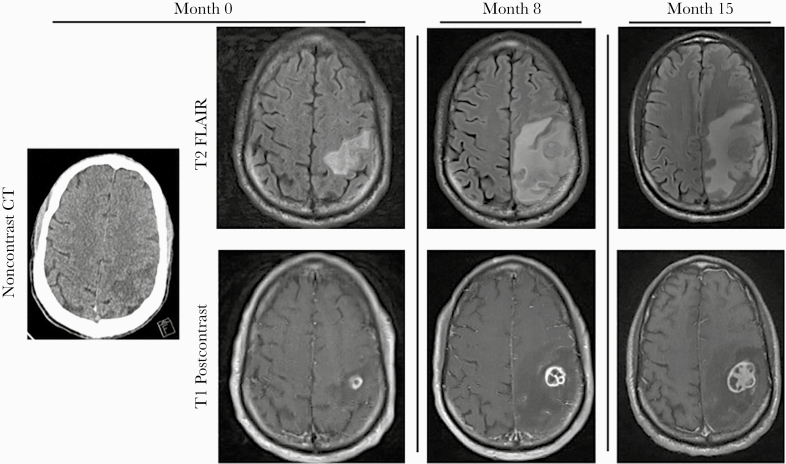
A, Noncontrast head CT, T2 FLAIR precontrast MRI, and T1-weighted postcontrast MRI from initial presentation. B, T2 FLAIR precontrast MRI and T1-weighted postcontrast MRI after discontinuing ART and MAC therapy. C, T2 FLAIR precontrast MRI and T1-weighted postcontrast MRI following completion of prednisone taper. Abbreviations: ART, antiretroviral therapy; CT, computed tomography; FLAIR, fluid-attenuated inversion recovery; MAC, *Mycobacterium avium* complex; MRI, magnetic resonance imaging.
